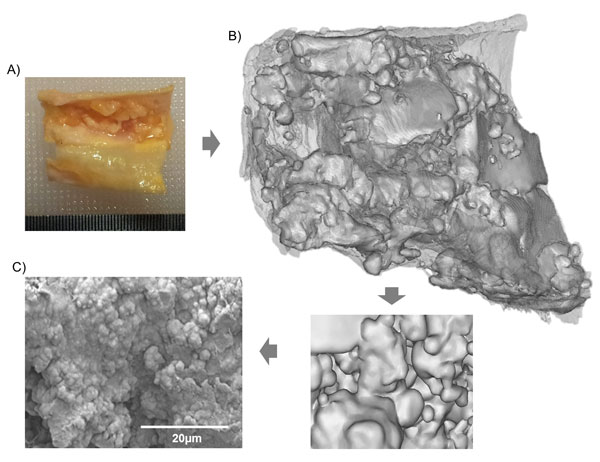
Characteristic imaging process of (A) advanced carotid plaque with (B) surface defects identified by μX-CT and (C) the surface topology further examined using SEM-EDX chemical analysis highlighting the irregular surface defects on the plaques luminal surface.
